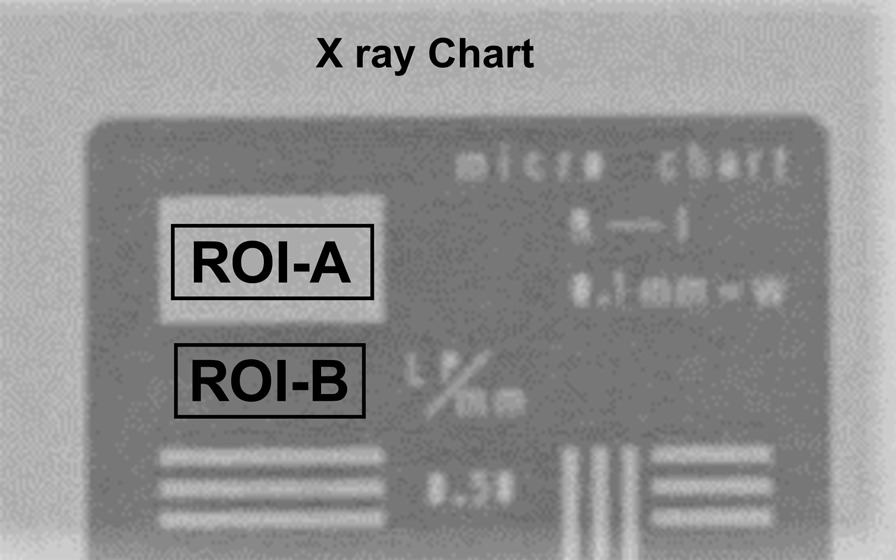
Region of interest (ROI) settings for contrast-to-noise ratio (CNR) measurements. Two ROIs were established on the X-ray chart
